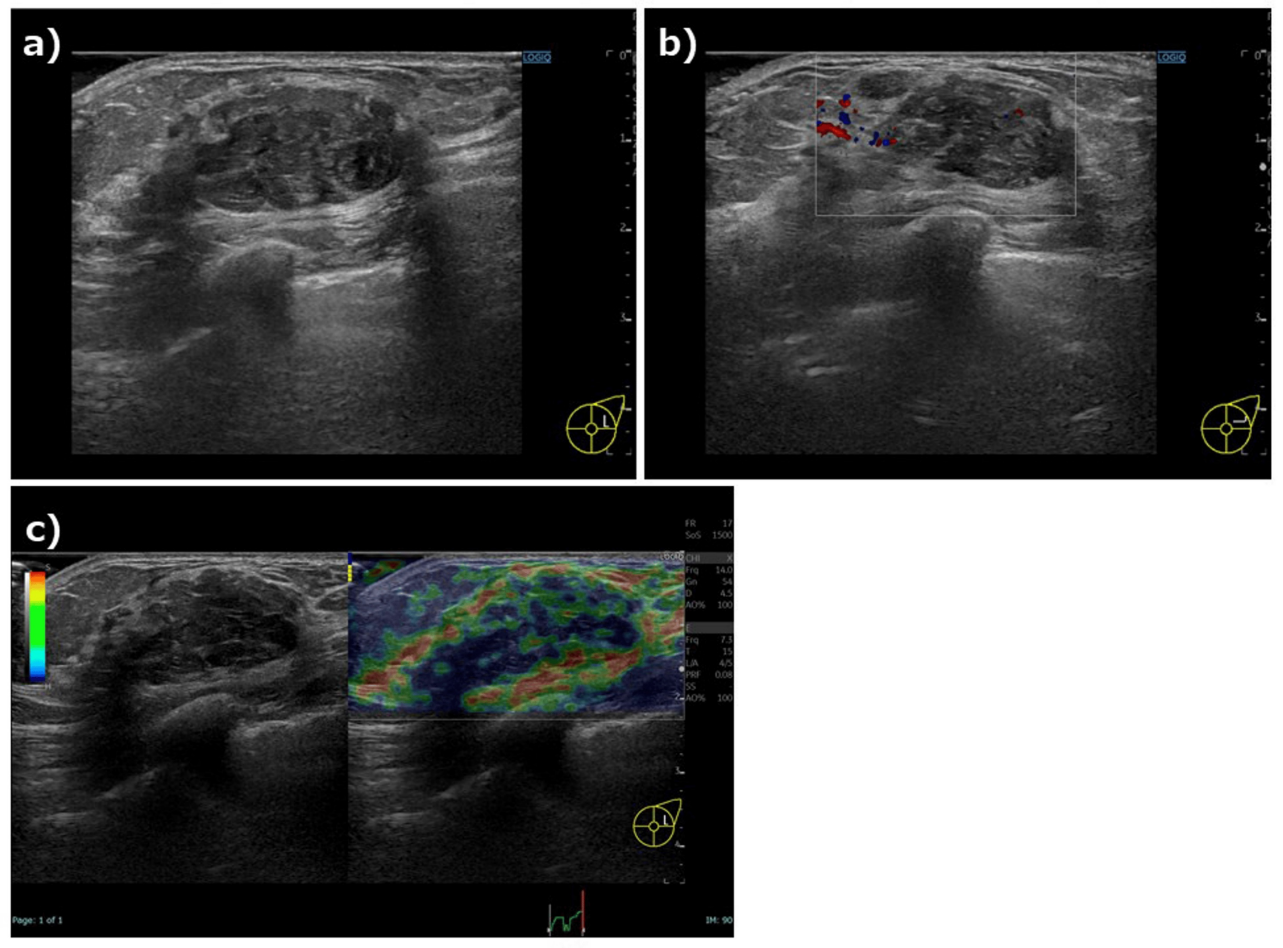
Ultrasonography findings in this case. Ultrasonography reveals a 26-mm well-defined hypoechoic mass in the upper outer area of the left breast (a). Power doppler ultrasonography shows internal blood flow within the mass (b), and elastography shows decreased strain (c).
